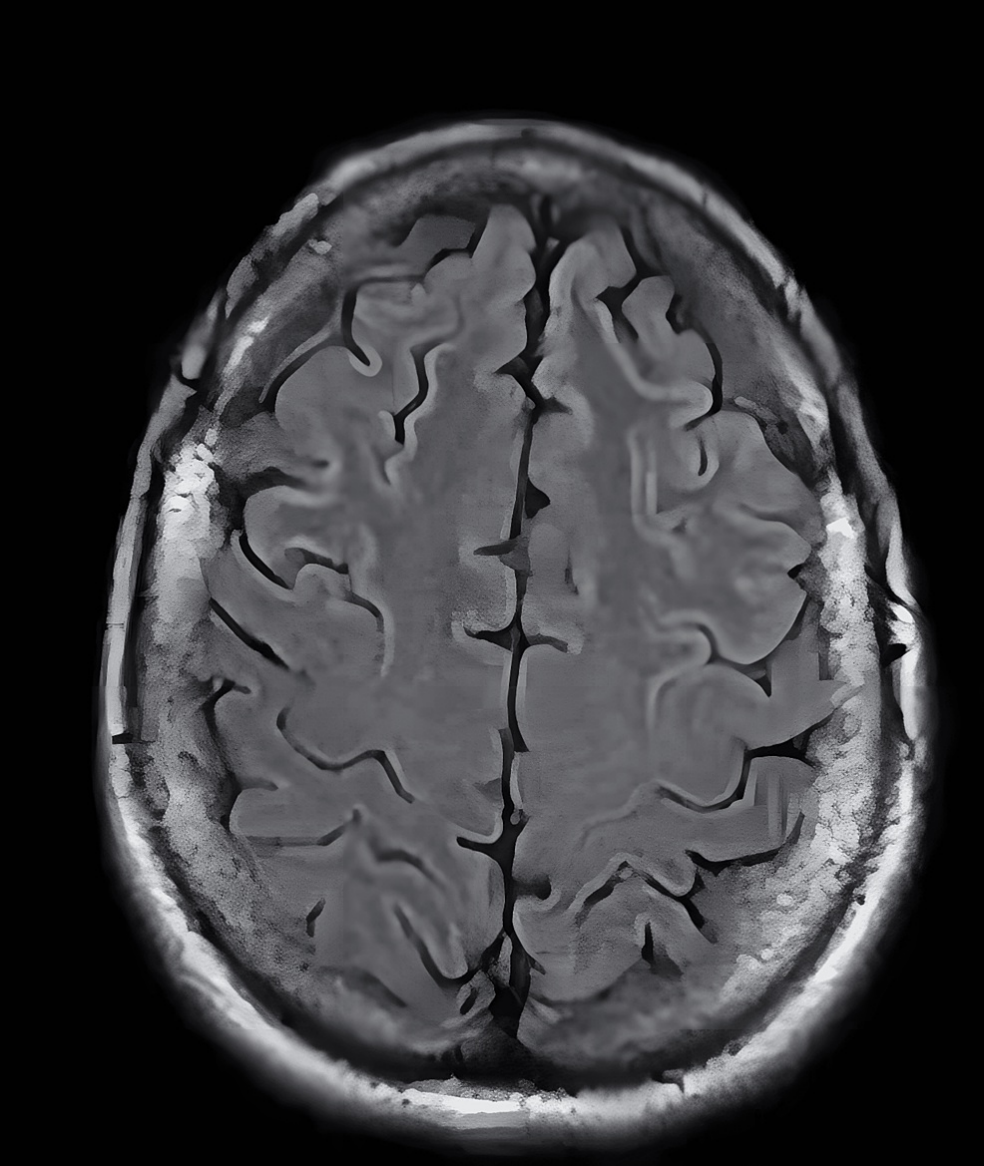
MRI of the brain reveals symmetric abnormal restricted diffusion and signal abnormality involving the cortex bilaterally MRI: magnetic resonance imaging
